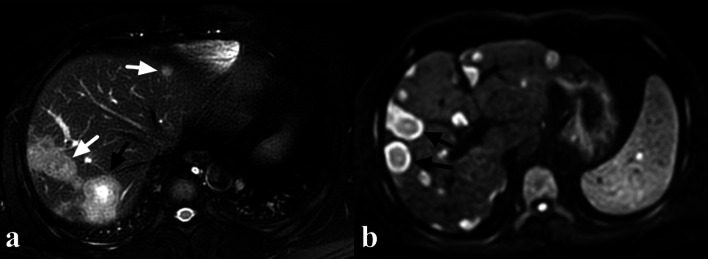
A 38-year-old male HEH patient. a On fat-saturated T2 weighted image, target sign could be observed on a medium lesion (black arrow), while both small and large lesions failed to show target appearance (white arrow). b Target sign on DWI image (800 b-value), consisted of concentric layers including relatively hyperintense in central tumor regions and peripheral zones which were markedly hyperintense relative to liver parenchyma (arrows)
